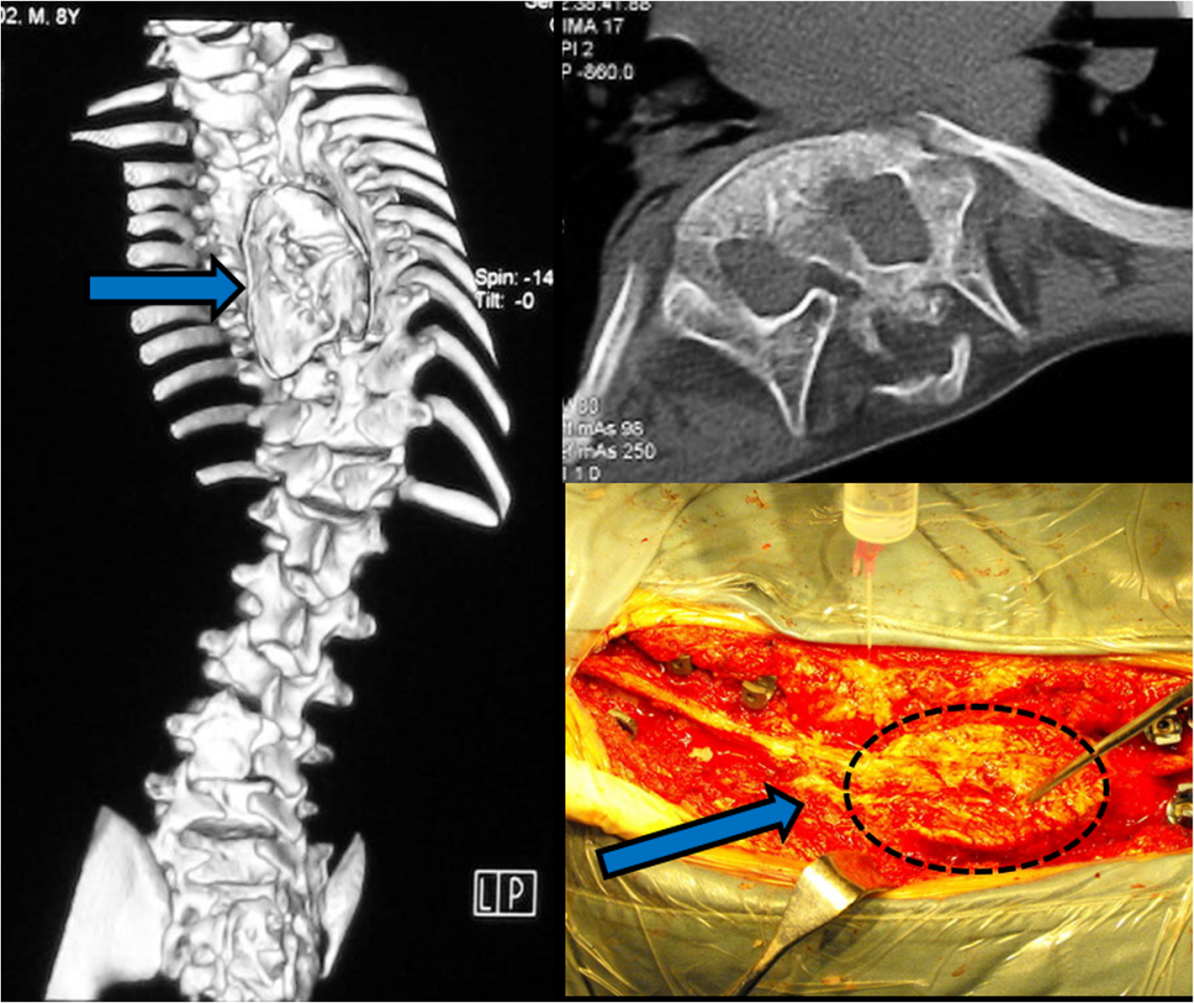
Images of an eight-year-old male patient diagnosed with congenital scoliosis and the type I split cord malformation. Three-dimension CT showed hypertrophic lamina and bulbous spinous processes in the middle thoracic region. These exuberant neural arches can be described as the “volcano-shape” deformity (with arrows in the figure)
